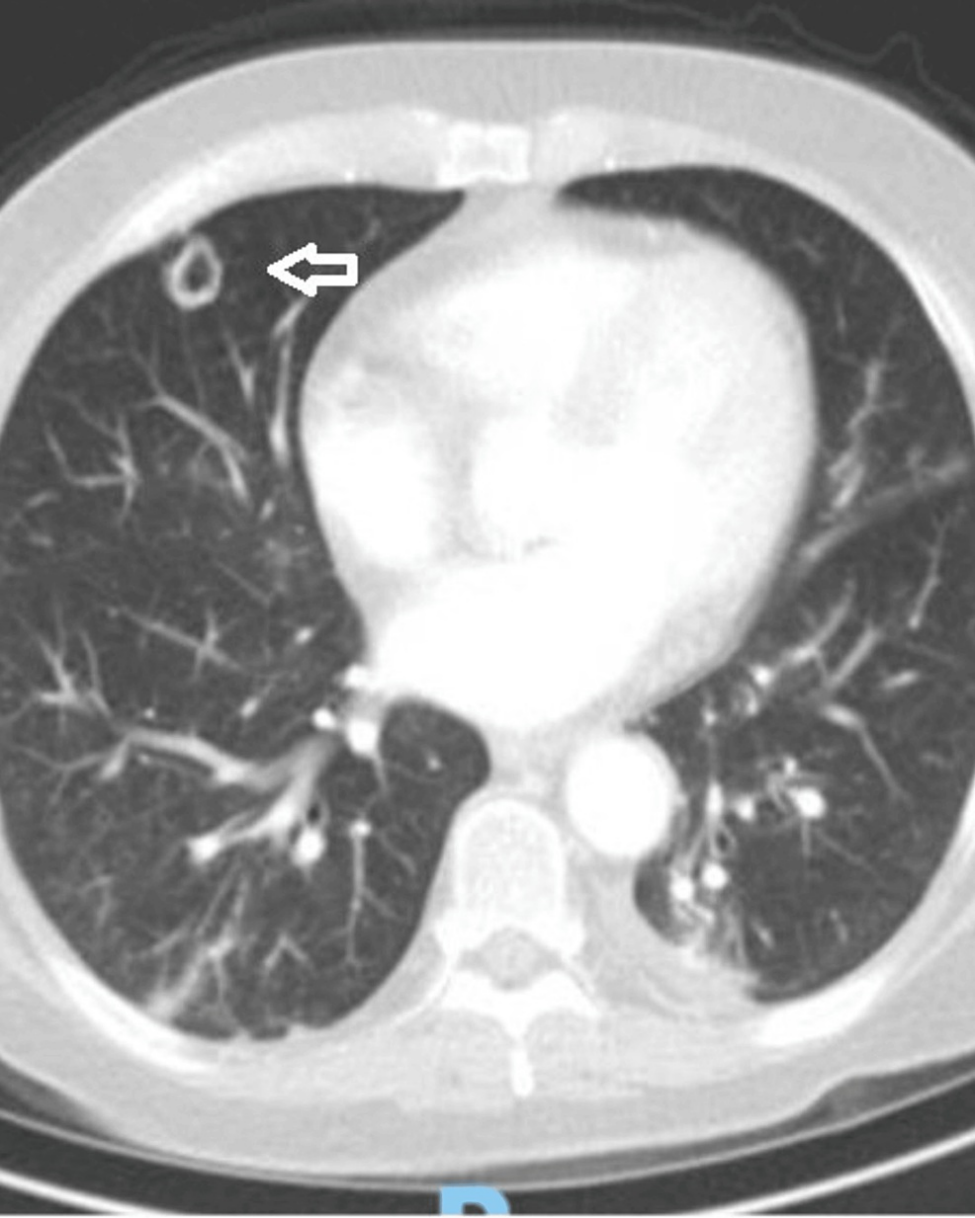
CT chest scan without contrast A computed tomography (CT) scan of the chest showed a 14-mm cavitary lesion in the middle lobe of the right lung (arrow).
